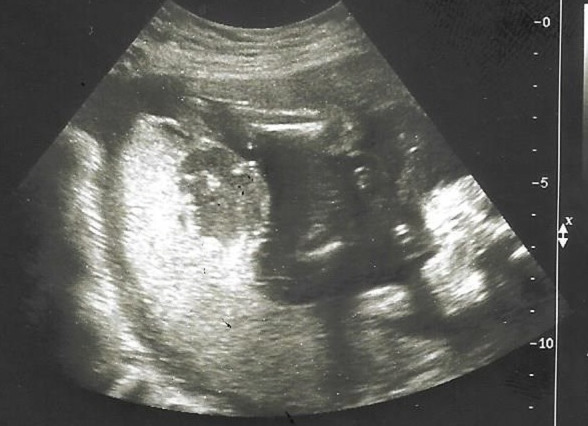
the placental chorioangioma on ultrasound at 21 weeks´ gestation
